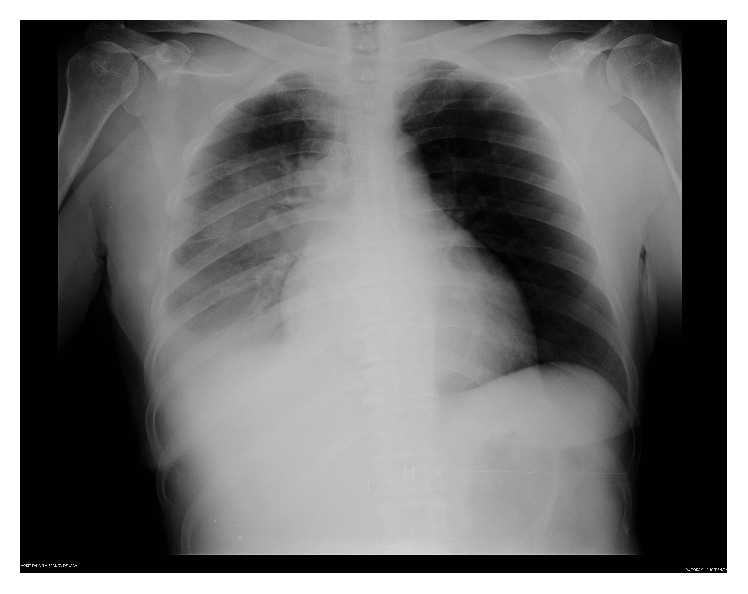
Admission chest radiograph showing patchy peribronchial and right lower lobe consolidation.
